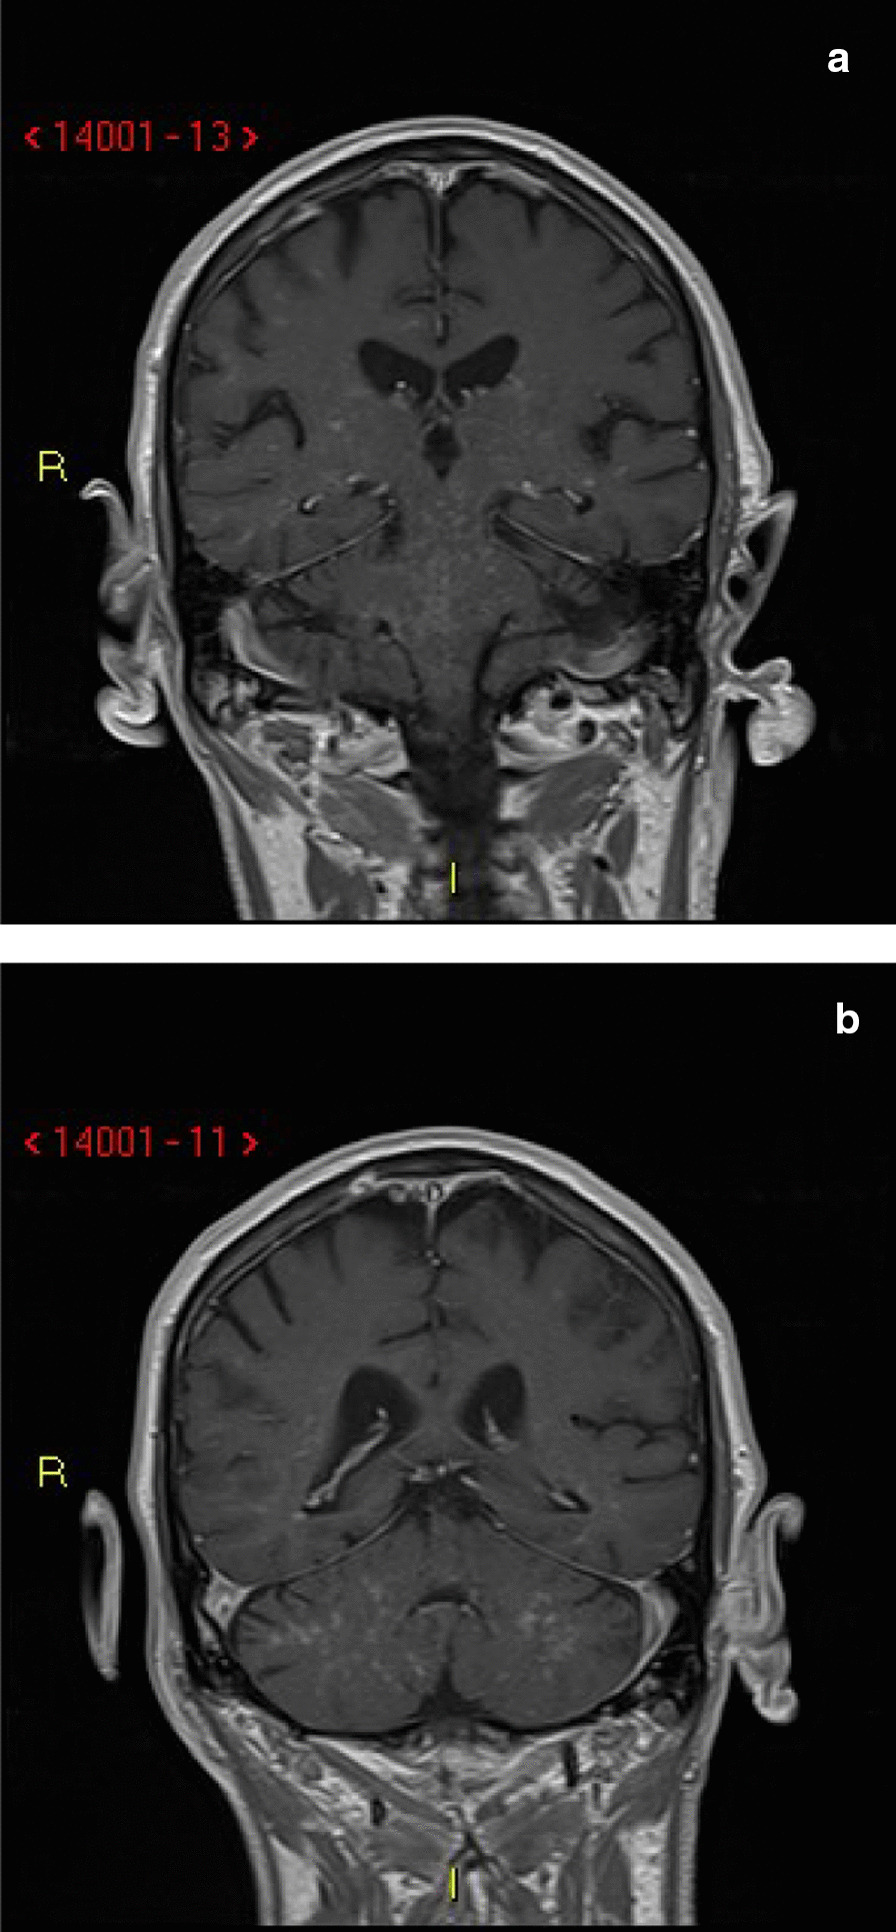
a, b Coronal magnetic resonance imaging T1 postcontrast images demonstrating angiocentric enhancement predominantly in the brainstem and cerebellum with involvement of the basal ganglia, thalami, and supratentorial white matter
